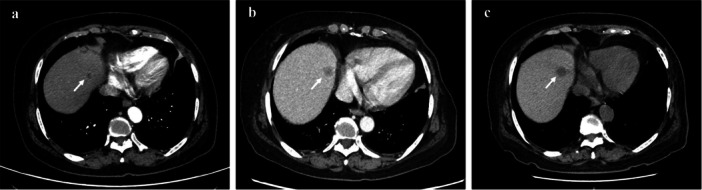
CT scan of the abdomen. (a) CT scan of the abdomen showed a small low‐density nodule with ring enhancement (0.8 cm in diameter) in the S8 segment of the liver. (b) CT scan of the abdomen showed the low‐density nodule in the S8 segment of the liver had increased in size (1.4 cm in diameter). (c) CT scan of the abdomen showed no significant change in the low‐density nodule (1.5 cm in diameter) in the S8 segment of the liver.
